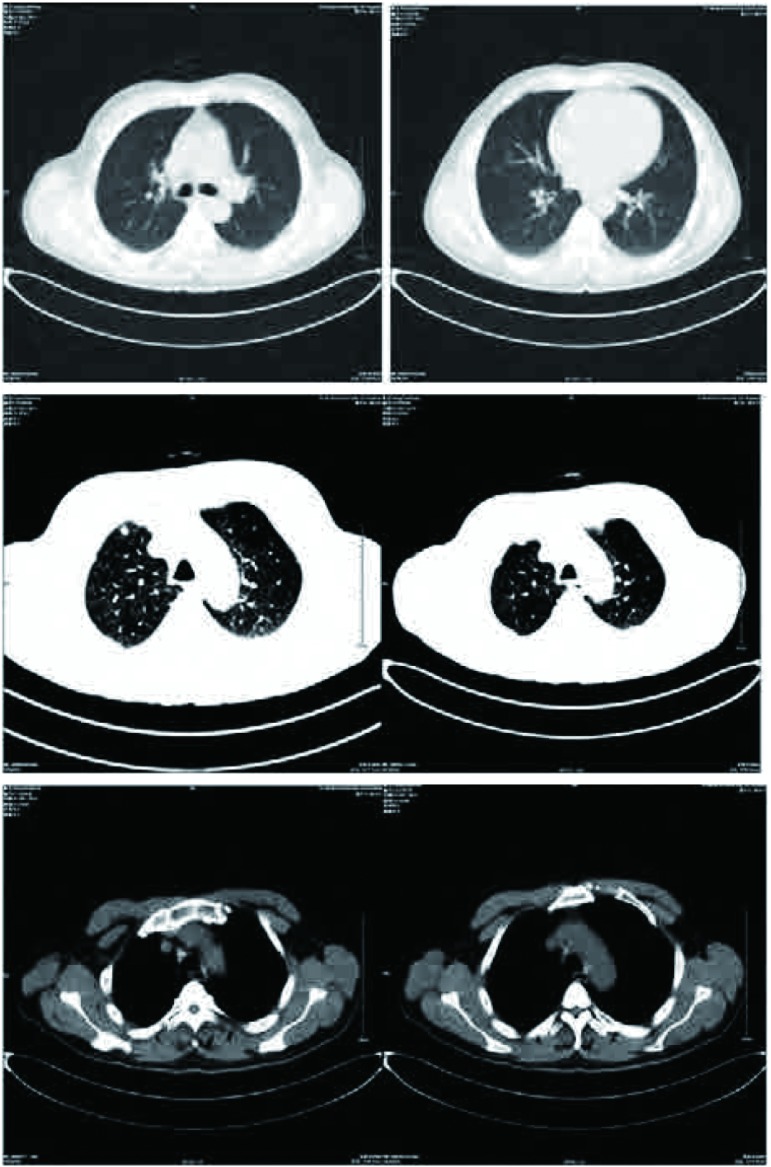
肺部高分辨CT示右肺下叶肺大泡，左肺上叶后段胸膜下小结节，纵隔及双肺门多发趋向钙化淋巴结，双侧胸膜增厚。 Chest CT scan demonstrated bullae in right lung lower lobe, a tubercle in posterior segment in left lung superior lobe, calcification lymph nodes in interpleural space and both hilus of lung and thickness in both membrana pleuralis.
